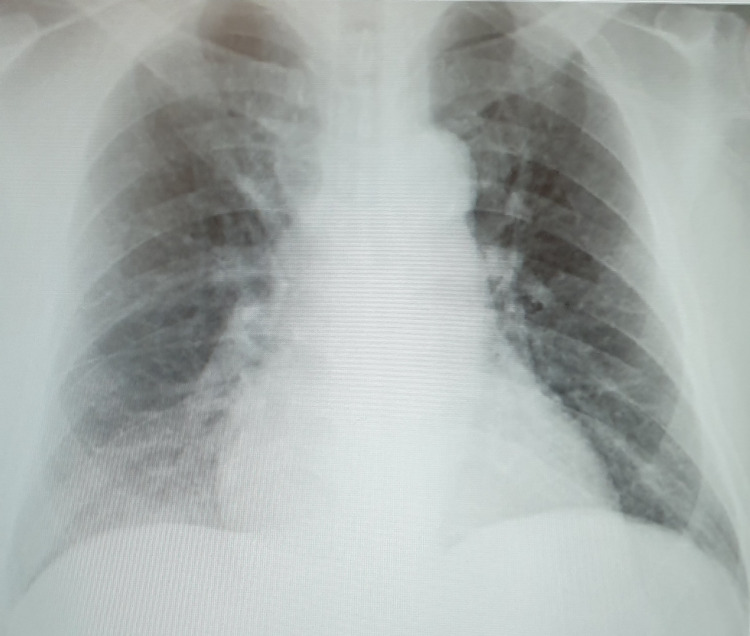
Chest X-ray shows cardiomegaly and pulmonary vascular congestion
